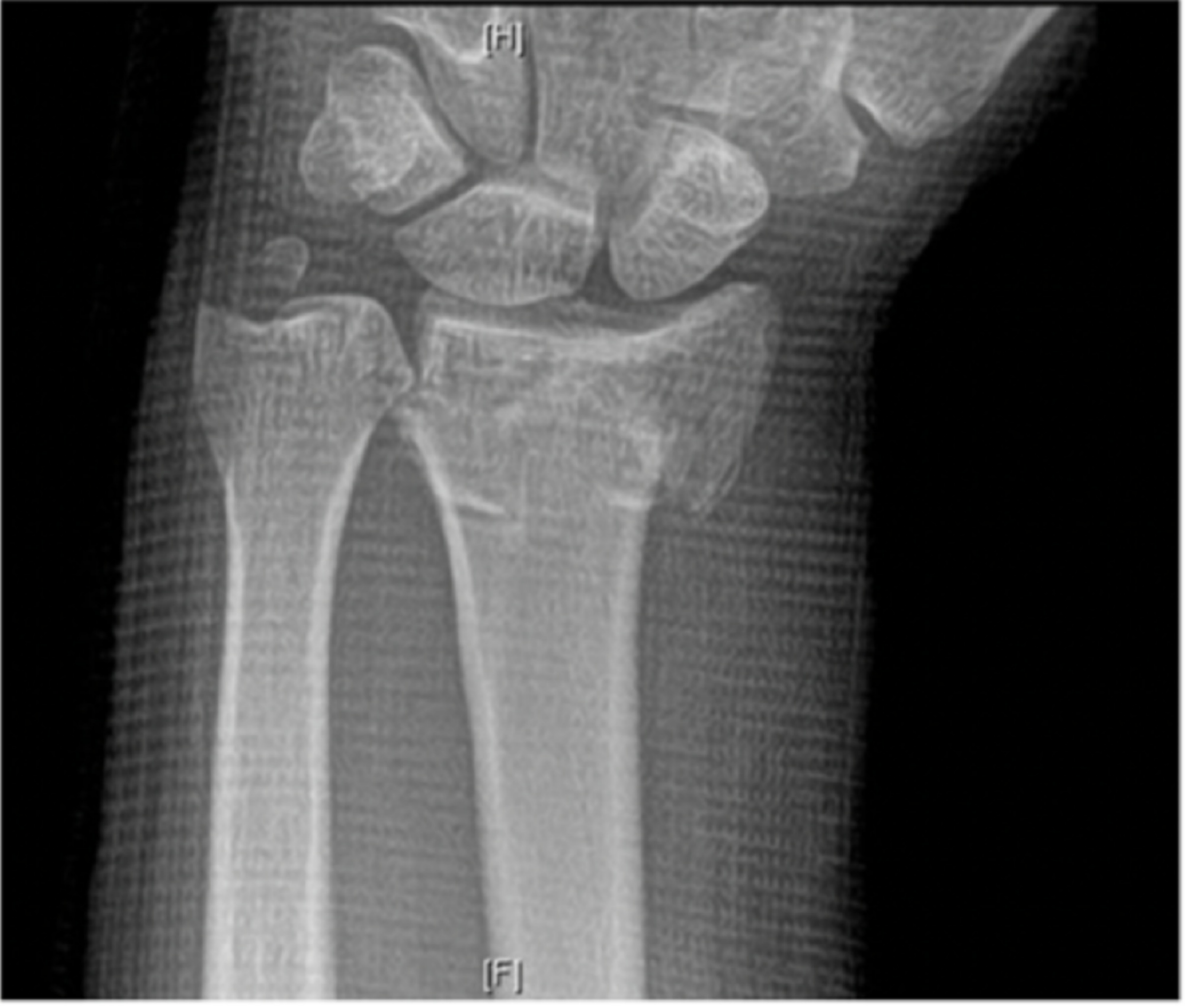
Anterior-posterior (AP) X-ray of the left wrist showing comminuted distal radius fracture with dorsal angulation of the distal fragment and a displaced ulnar styloid fracture
